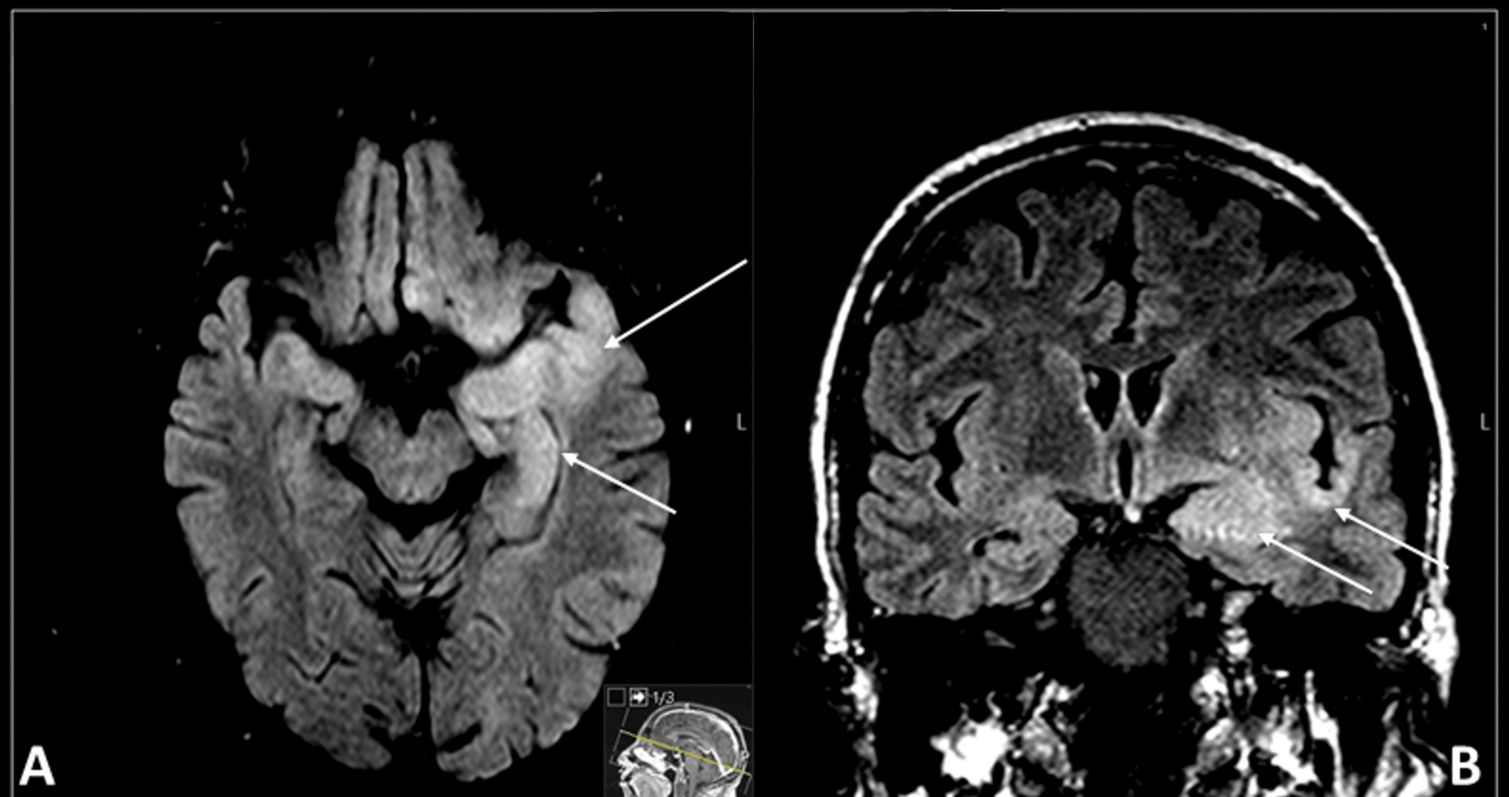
Brain MRI showing parenchymal hyperintensity predominantly visible on FLAIR sequences, consistent with limbic encephalitis. (A) Axial FLAIR image highlighting hyperintensity in the left hippocampus and amygdala (arrows), with mild cortical swelling. (B) Coronal FLAIR image demonstrating hyperintensity in the left insular cortex and anterior temporal cortex (arrows) MRI: magnetic resonance imaging, FLAIR: fluid-attenuated inversion recovery
